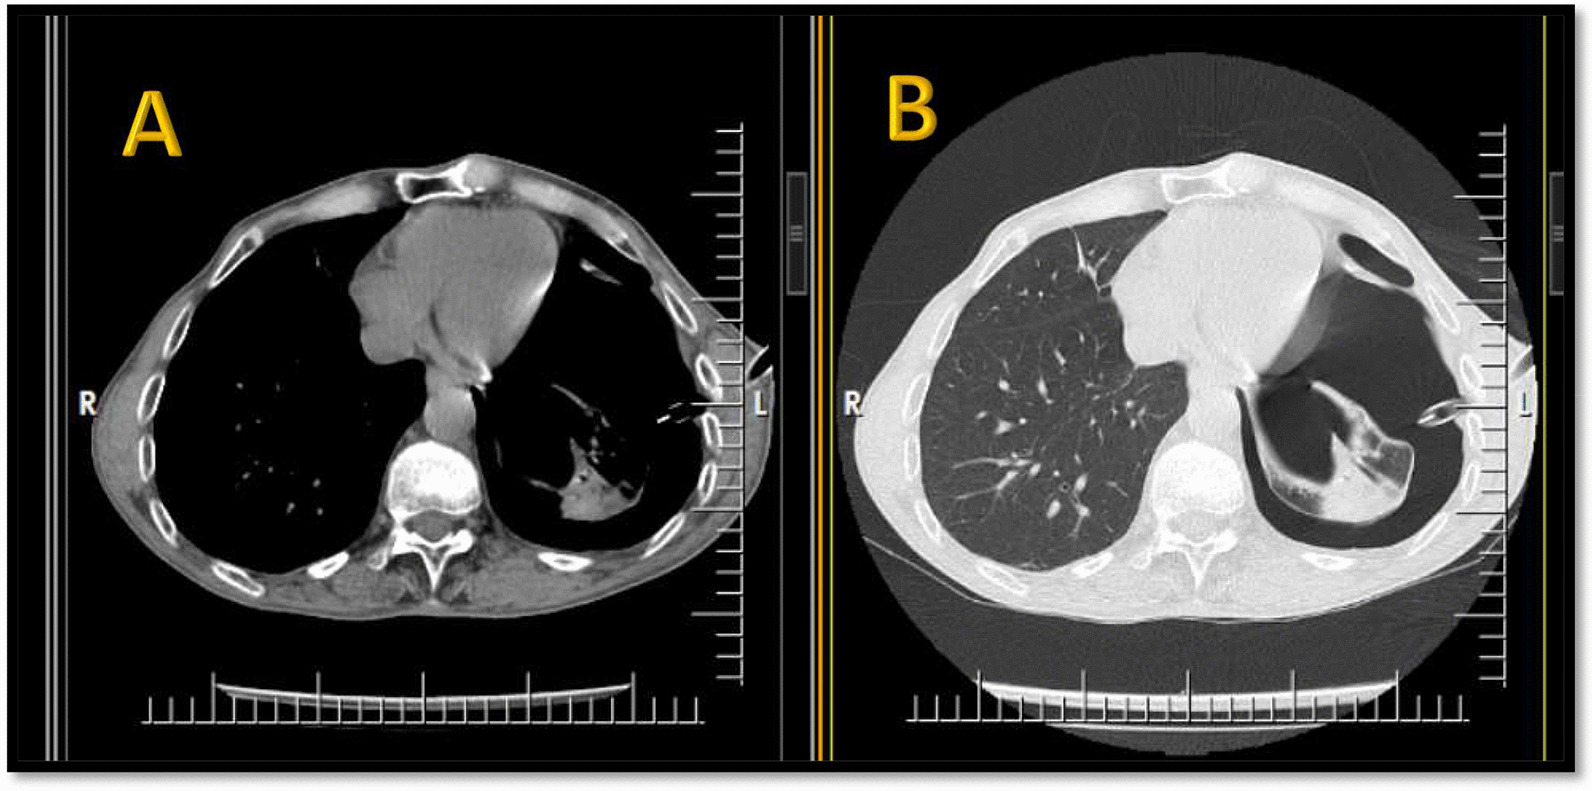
Spiral chest CT scan without contrast (2nd admission), not-resolved left sided pneumothorax after the chest tube insertion, a mediastinal axial view, b lung window axial view
